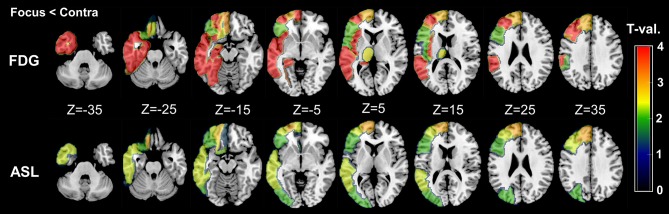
Regional maps of *t*-values (absolute values) in side-specific differences. Only significant VOIs at *p* < 0.05 without Bonferroni correction are shown. For easy understanding, the scale of *t*-values is unified between 0 and 4. However, it should be noted that a few VOIs in FDG-PET showed over 4–5 in the absolute *t*-values (please see also Table 3).
